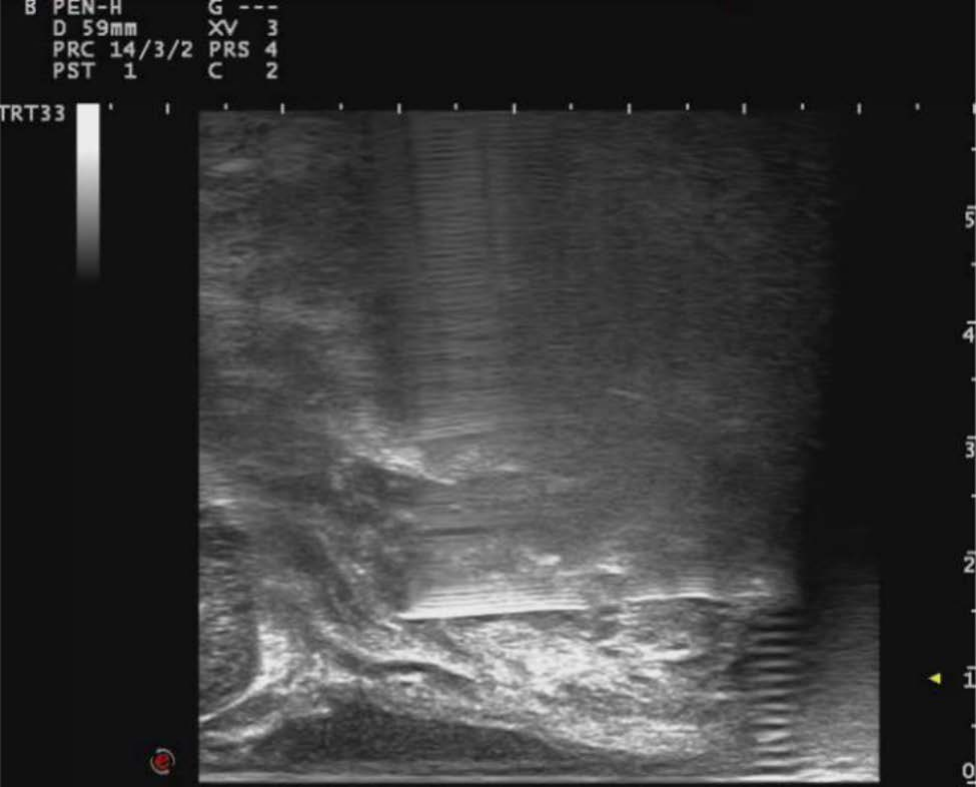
Ultrasound-guided anesthesia for injection of local anesthetics into the puborectal muscle. Under ultrasound guidance, we can evenly inject local anesthetic drugs into the anterior and posterior capsule of the puborectal muscle, and effectively avoid the larger venous plexus in the pelvic floor, reducing the probability of side effects of local anesthetic drugs.
